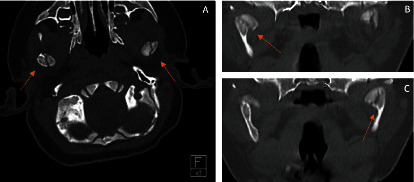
Initial CT scan in axial view (A). Coronal view of right condylar fracture (B). Coronal view of left condylar fracture (C).
